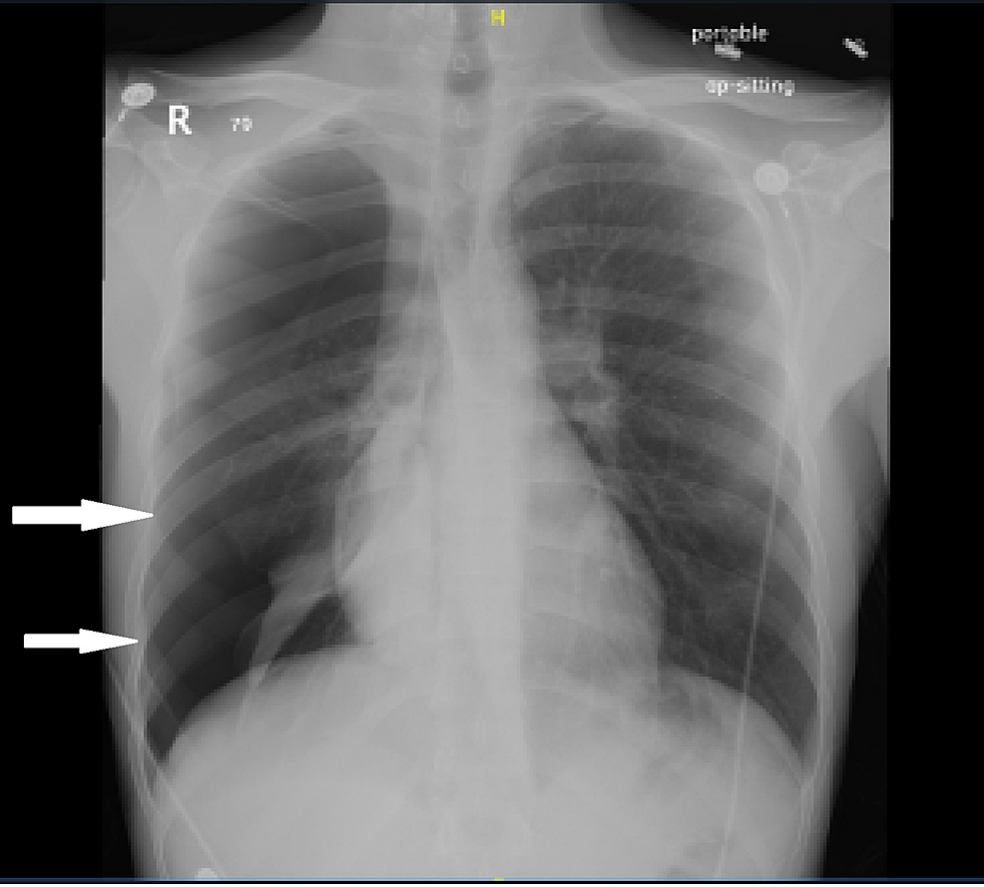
Chest X-ray showing collapsed lung and pneumothorax (white arrows)
